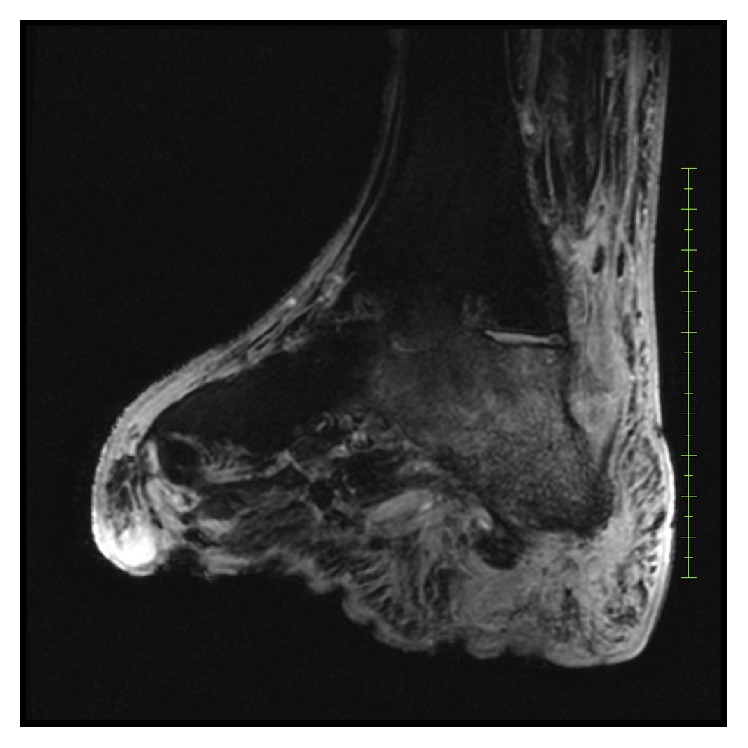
Magnetic Resonance Imaging demonstrating chronic osteomyelitis of the right calcaneus.
